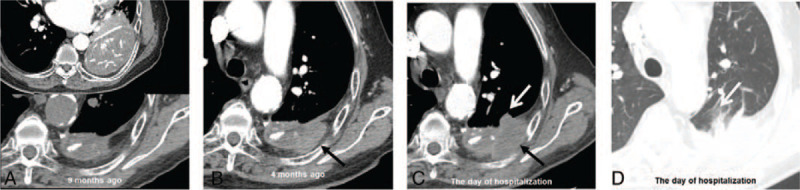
Computed tomography (CT) of the chest at different times. (A) Contrast enhanced CT scan showed a chronic empyema cavity with a peripheral rim and dystrophic calcifications in the left lower hemithorax. (B) Chest CT images taken 4 months ago showed a lenticular mass (black arrow) at the edge of the chronic empyema (3.7 cm in size) which was newly occurring compared with prior CT. (C, D) Follow-up chest CT images taken at admission showed a remarkable enlargement of the prior pleural soft tissue mass with extension to the adjacent chest wall (black arrow) and invasion of the lung parenchyma (white arrow).
